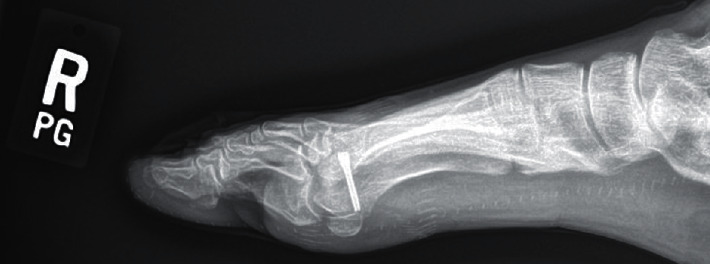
Postoperative lateral radiograph of the right foot demonstrating hallux rigidus correction with OCA implantation on the metatarsal head.
